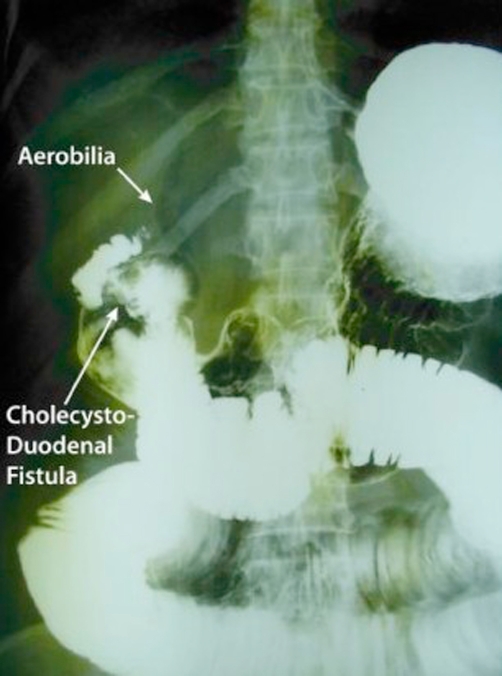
Roentgenogram of the barium follow through showing evidence of small bowel obstruction (dilated bowel loops) with aerobilia and the cholecysto-duodenal fistula.
